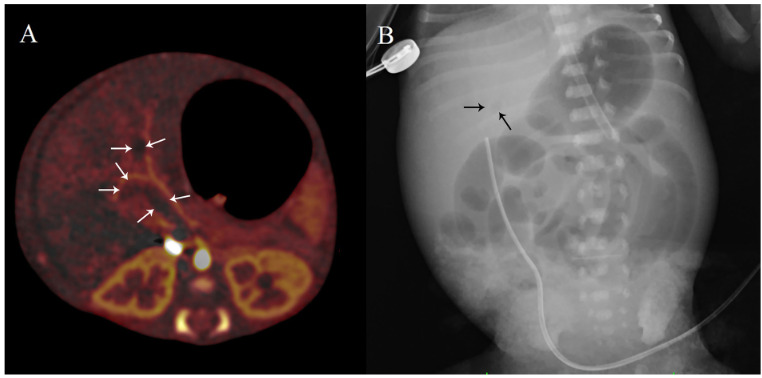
Axial contrast-enhanced image (A) of a preterm infant showing portal thrombus (see white arrows). The radiolucent area (see black arrows) on the direct abdominal X-ray (B) was misdiagnosed as PVG.
